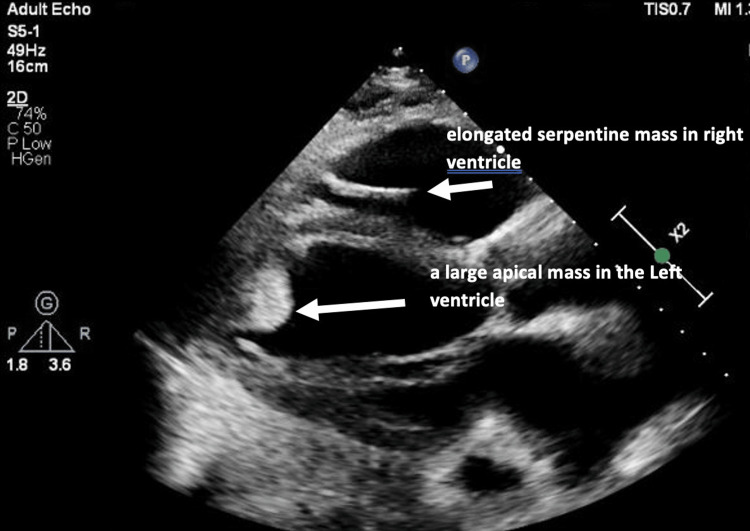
Transthoracic echocardiogram parasternal long-axis view showing a large apical mass in the LV measuring 3.5 × 3 cm (arrow) and elongated serpentine mass in the RV (arrow). LV: left ventricle, RV: right ventricle
